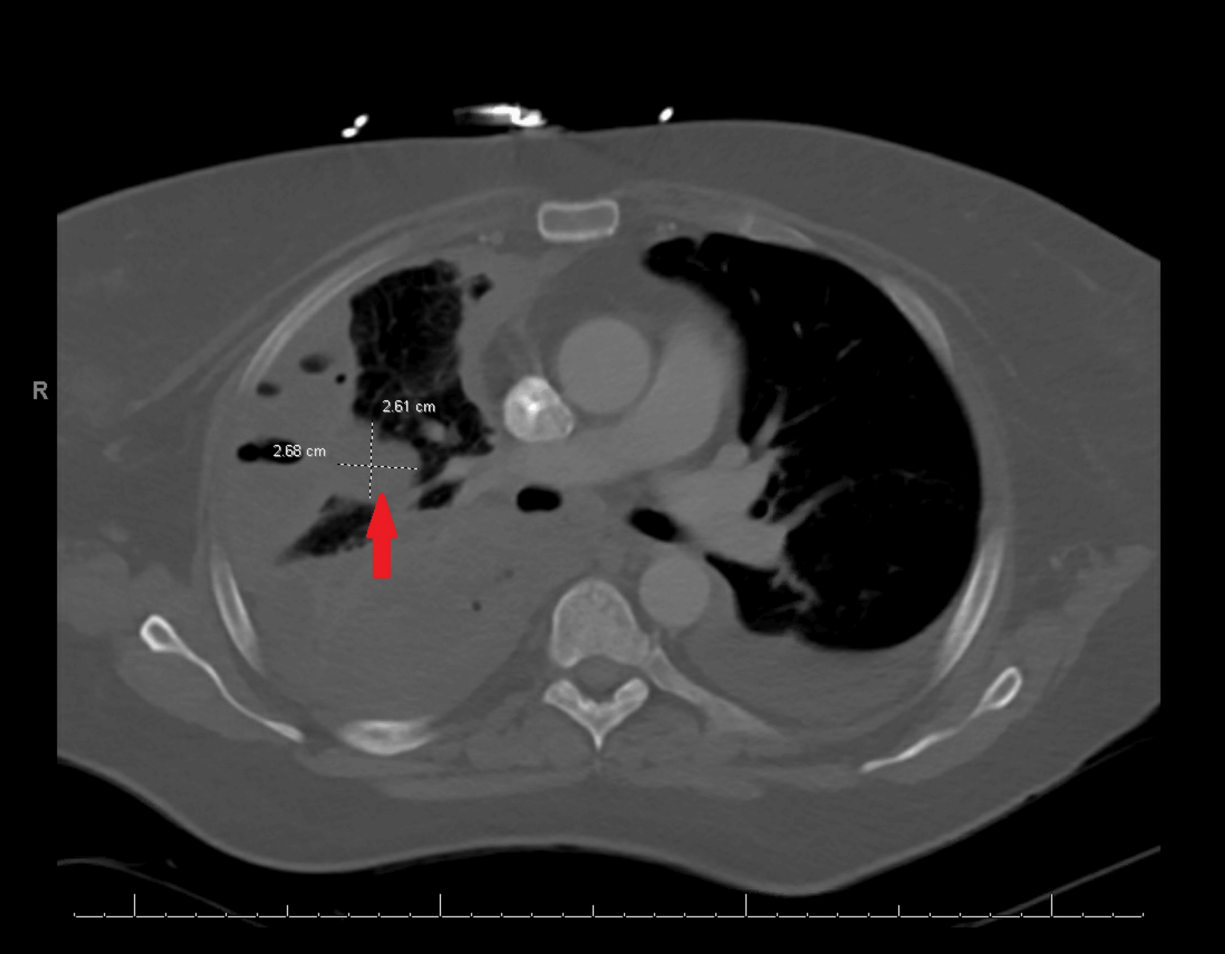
A computed tomography angiography of the patient’s chest showing a large right hydrothorax with a mediastinal shift to the left and a poorly defined mass-like right lung consolidation (arrow).
